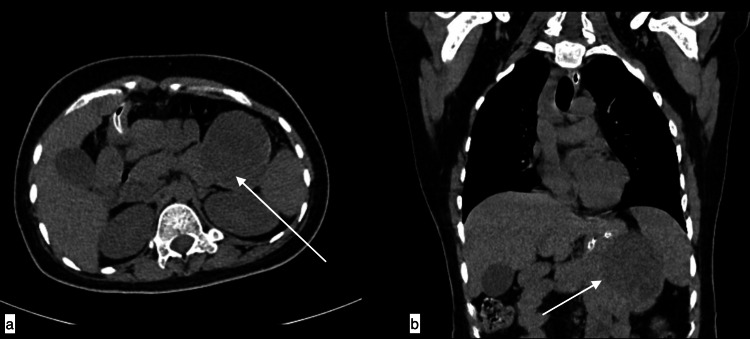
Pancreatic hydatid cyst A 24-year-old female with pancreatic hydatid cyst presented with fever, cough, and shortness of breath for two months. Axial (a) and coronal (b) non-contrast CT abdomen revealed a well defined thin walled hypodense lesion of fluid attenuation with wall calcification measuring 6.5x6x6 cm noted along the distal body and tail of pancreas extending into lesser sac, laterally abutting the splenic hilum with few internal septations.
